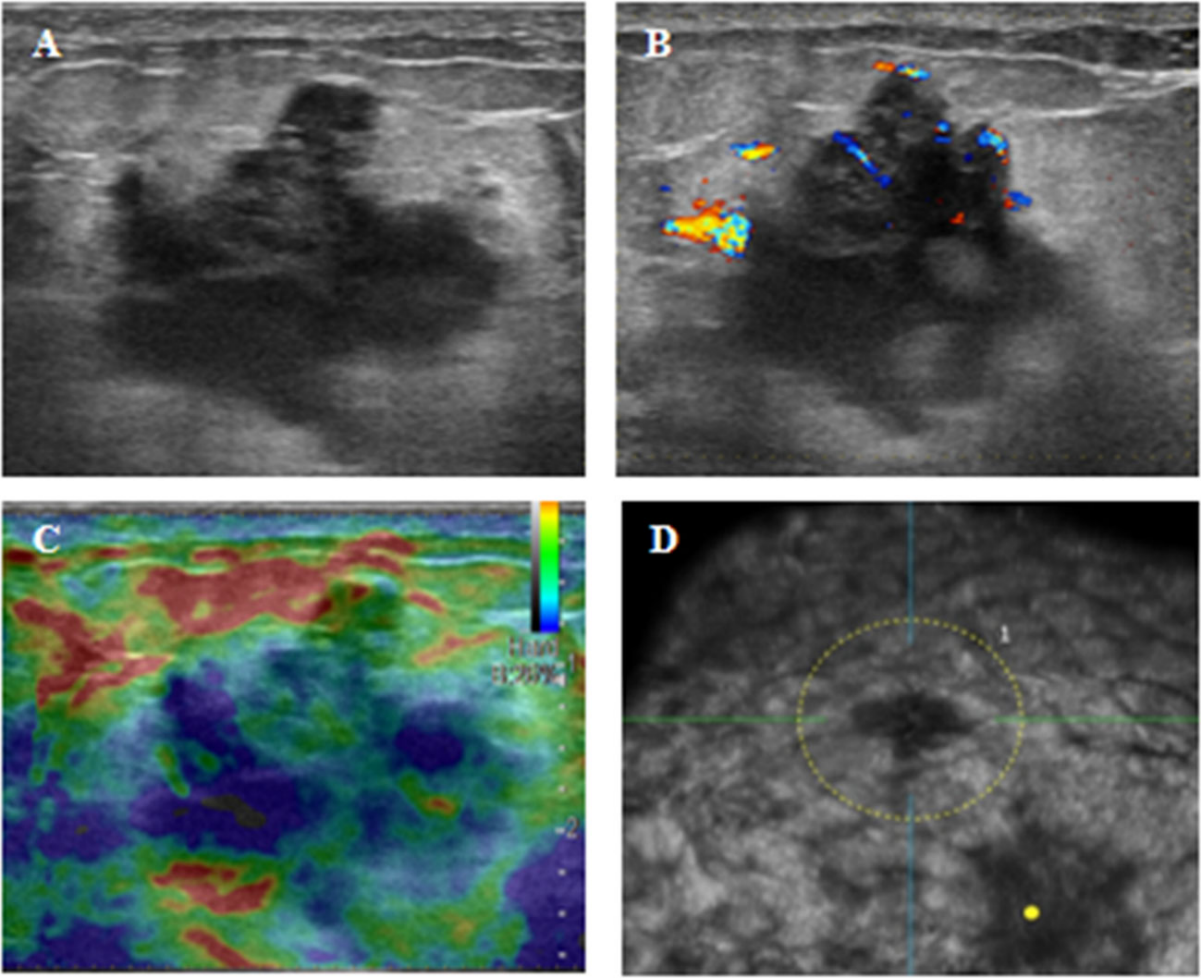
Ultrasound scan and automated breast ultrasound system (ABUS). (A) Conventional ultrasound showing a 29×21×20mm hypoechoic mass in the upper inner quadrant of the left breast, with irregular contours and heterogeneous echotexture; (B) CDFI displaying Grade II blood flow within the mass; (C) Strain elasticity image indicating a moderately firm mass texture; (D) ABUS revealing a “Convergence Sign” in the mass.
